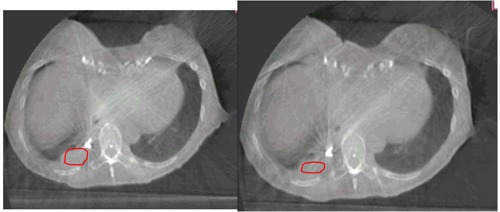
The CBCT image of the only lesion (Patient 2, tumor #2) for which the GTV (red line) volume difference was smaller on the image after the 3rd fraction compared to the image before the 1st fraction: image taken before SBRT (left) and image taken after treatment on Day 3 of SBRT (right). A prominent artifact, due to a metal clip, is detectable.
